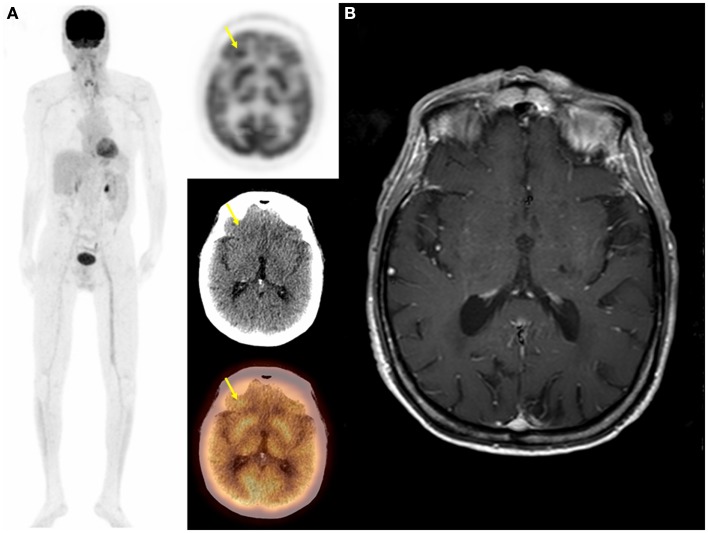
Seventy-one-year-old male with metastatic squamous cell carcinoma of the head and neck. On follow-up PET/CT: MIP, transaxial PET, CT, and fused images (A) demonstrated an unsuspected hypermetabolic focus is the right frontal lobe with no definite abnormality on CT in addition to metastatic head and neck cancer. Brain MRI was negative for metastasis (B). In this case PET was falsely positive and the focal uptake in the right frontal lobe was thought to be due to artifact.
